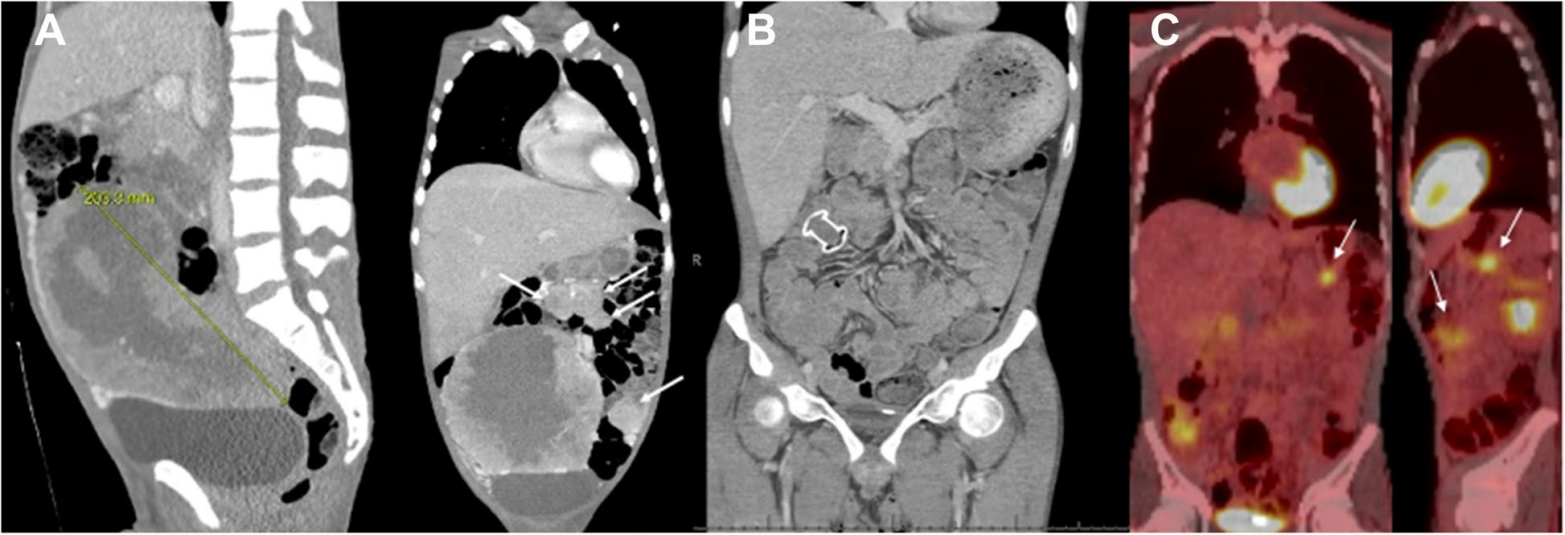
Radiographic image findings. A Sagittal and coronal CT abdominal images on initial presentation showing a large abdominopelvic mass. White arrows in coronal view highlight metastatic disease in the abdomen. B Coronal CT abdominal post-surgical interventions showing absence of the main mass and the LAMS stent. C Coronal and sagittal PET CT images one month post initiation of nab-sirolimus showing stable residual disease in the abdomen as highlighted by the white arrows
